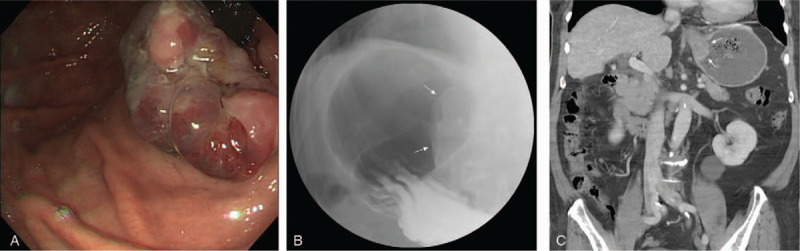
(A) EGD revealed a tumor at the cardia side of the esophagogastric junction with dark-red and gray pigmentation. (B) Upper gastrointestinal series indicated a nearly 4-cm submucosal mass at the gastric cardia. (C) CT indicated a mass with rim enhancement. CT = computed tomography, EGD = Esophagogastroduodenoscopy.
